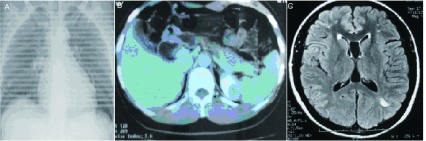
肿瘤原发及转移部位影像学资料。A：CT示右肺门处一3 cm×4 cm团块影；B：腹部转移灶；C：脑部转移灶 Primary tumor and metastatic sites radiographic imagings. A: CT showing a 3 cm×4 cm clumps shadow on the hilum of lung; B: Abdominal metastasis; C: Brain metastasis
